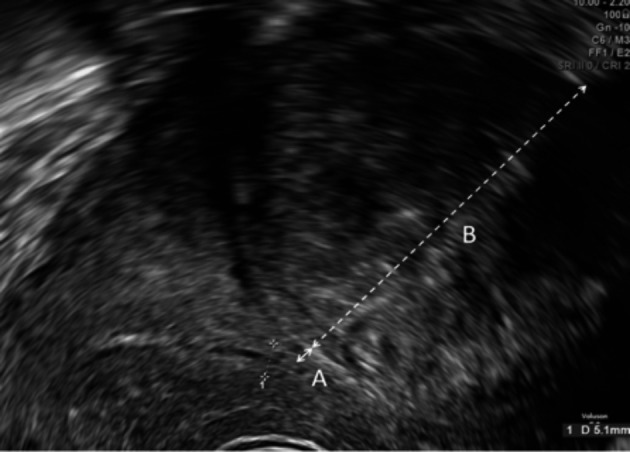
Ultrasound mage of an intramural/subserous fibroid showing the distance of the fibroid from the endometrial cavity (A) and the extra-cavity size (B).
